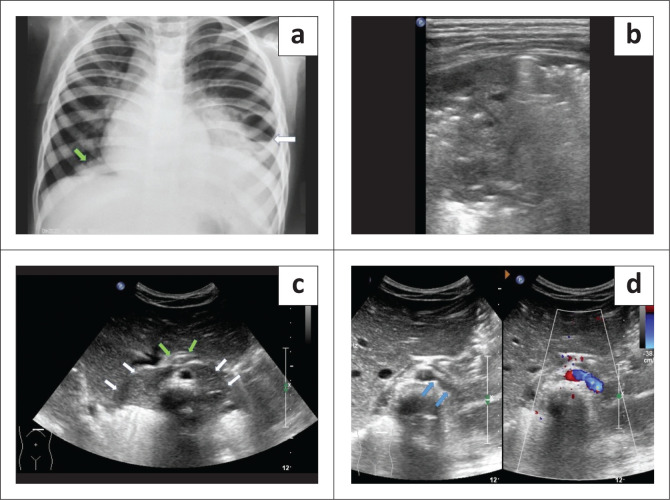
Bilateral intralobar pulmonary sequestration associated with true horseshoe lung in a 5-year-old girl with recurrent chest infections. (a) Frontal chest radiograph reveals consolidation with cavitation (white arrow) in the left mid and lower zones and patchy consolidation in the right lower zone, near the cardiophrenic angle (green arrow). (b) High frequency ultrasound image of the chest depicts the left lung consolidation. (c) Ultrasound image, transverse view through the epigastrium, shows abnormal soft tissue just above the diaphragm at the bases of both lower lobes (white arrows). This soft tissue is seen in continuity across the midline anterior to the aorta and posterior to the oesophagus (green arrows). (d) Transverse ultrasound image with colour doppler demonstrates an aberrant arterial branch (blue arrows) arising from the abdominal aorta and supplying the soft tissue, suggesting pulmonary sequestration.
